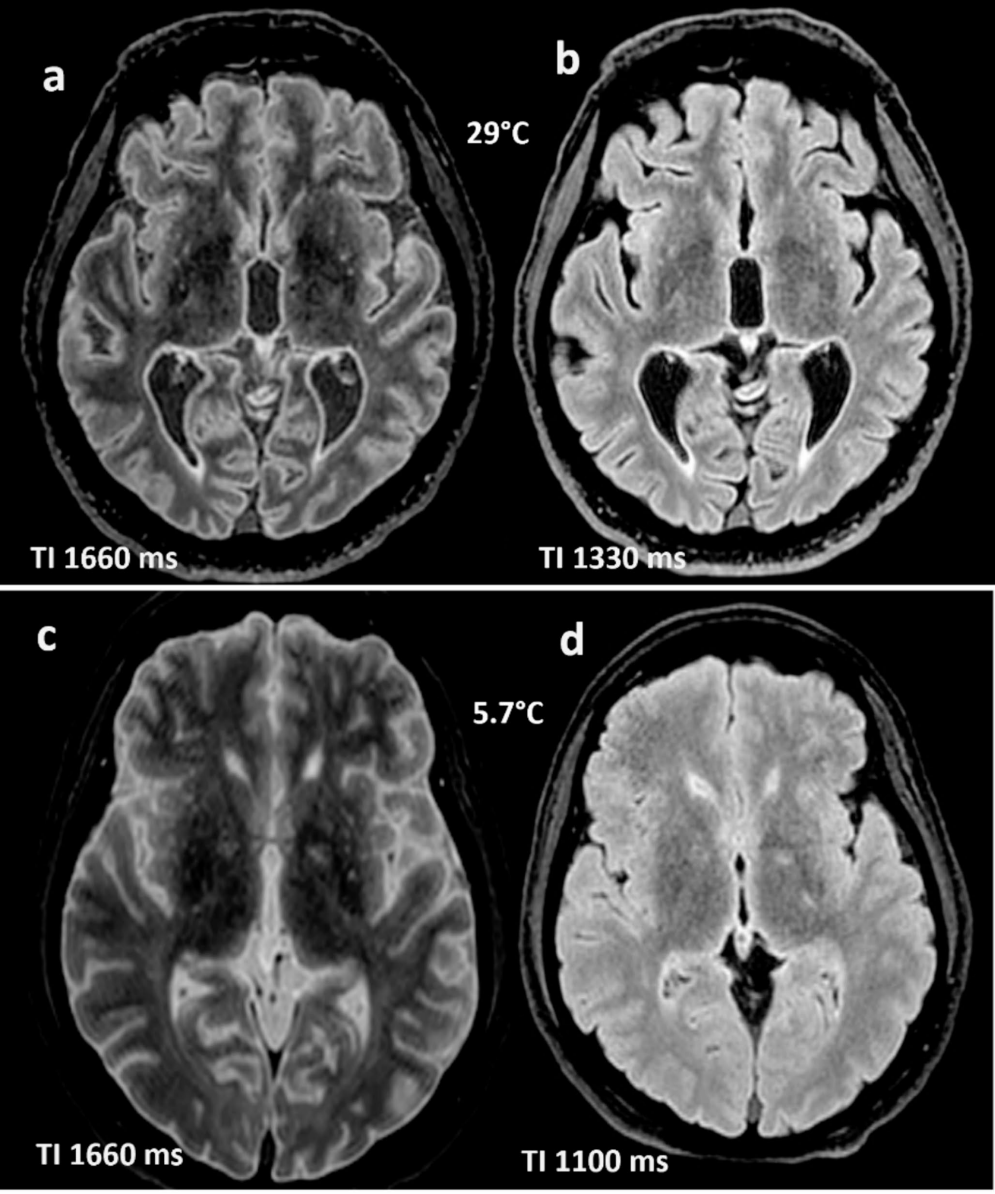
Example of axial FLAIR reconstructions of the brain with different TI. a) and b): same case at 29 °C; a: TI original 1660 ms and optimal b: TI 1330 ms; c) and d): same case at 5.7 °C; c: TI original 1660 ms and optimal d: TI 1100 ms
